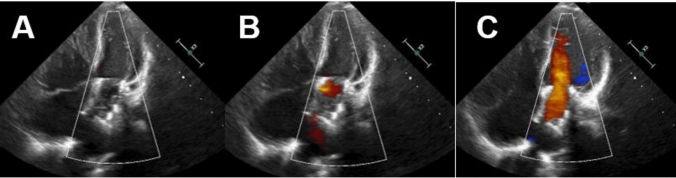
Follow-up echocardiography 2 years after the procedure. A Apical 4 C Melody valve. B Apical 4 C in diastole with Melody valve with adequate leaflet closure. C Apical 4 C with color Doppler without evidence of significant flow acceleration, with a mean valvular gradient of 5.5 mmHg
